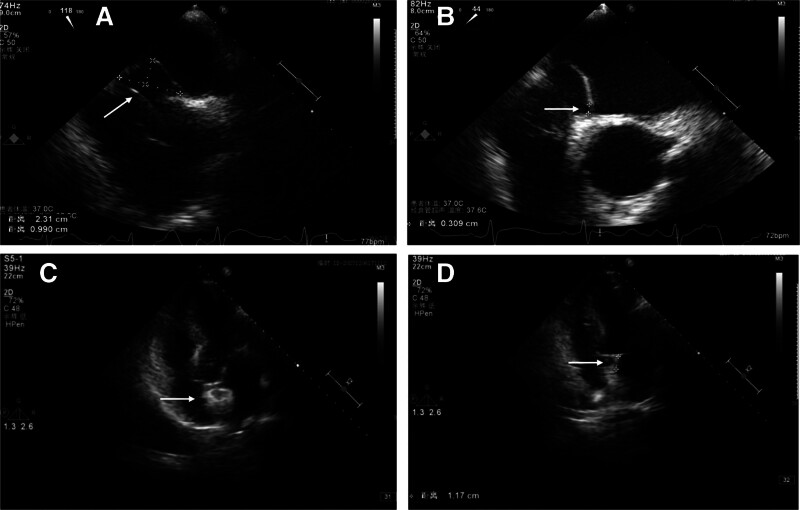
Transesophageal echocardiography (TEE) and ultrasound-guided foramen ovale interventional closure surgery. (A) TEE showing atrial septal bulge (23.1 mm × 9.9 mm, white arrow). (B) Patent foramen ovale (3.1 mm, white arrow). (C) Amplatzer occluder device (white arrow). (D) The distance between the occluder and the mitral valve root is 11.7 mm (white arrow).
